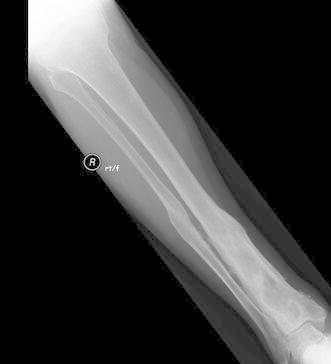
Pre-operative plain radiograph of the right distal tibal in a 57 year old male with chronic post-traumatic osteomyelitis distal tibia
